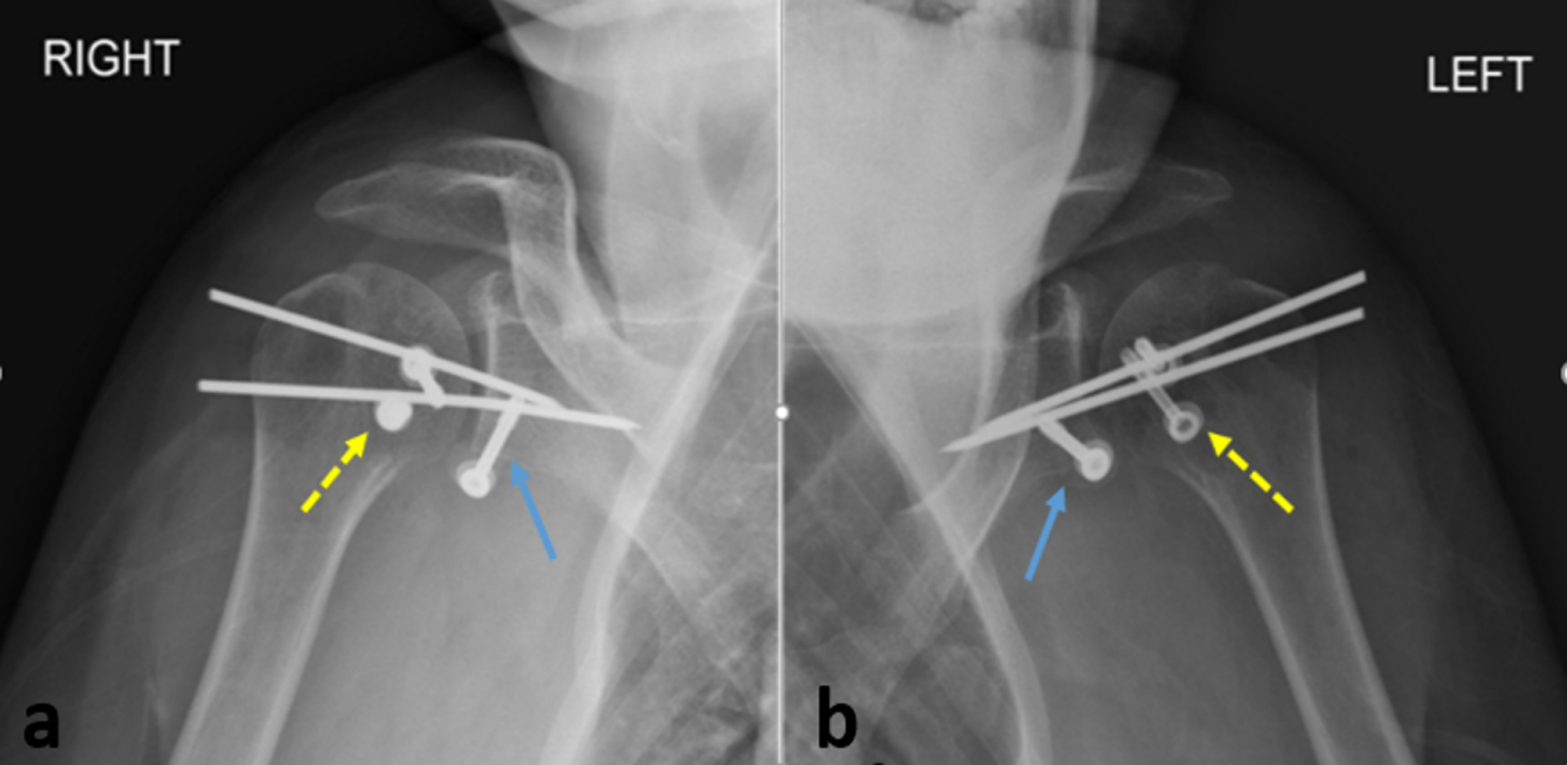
(a, b) Postoperative antero-posterior radiographs of both shoulders showing fixation of the coracoid fragment to the antero-inferior glenoid rim (blue arrow), with fixation of the lesser tuberosity osteotomy (yellow dashed arrow), and pin transfixion of the gleno-humeral joint. Shoulder joint is well reduced in both sides
